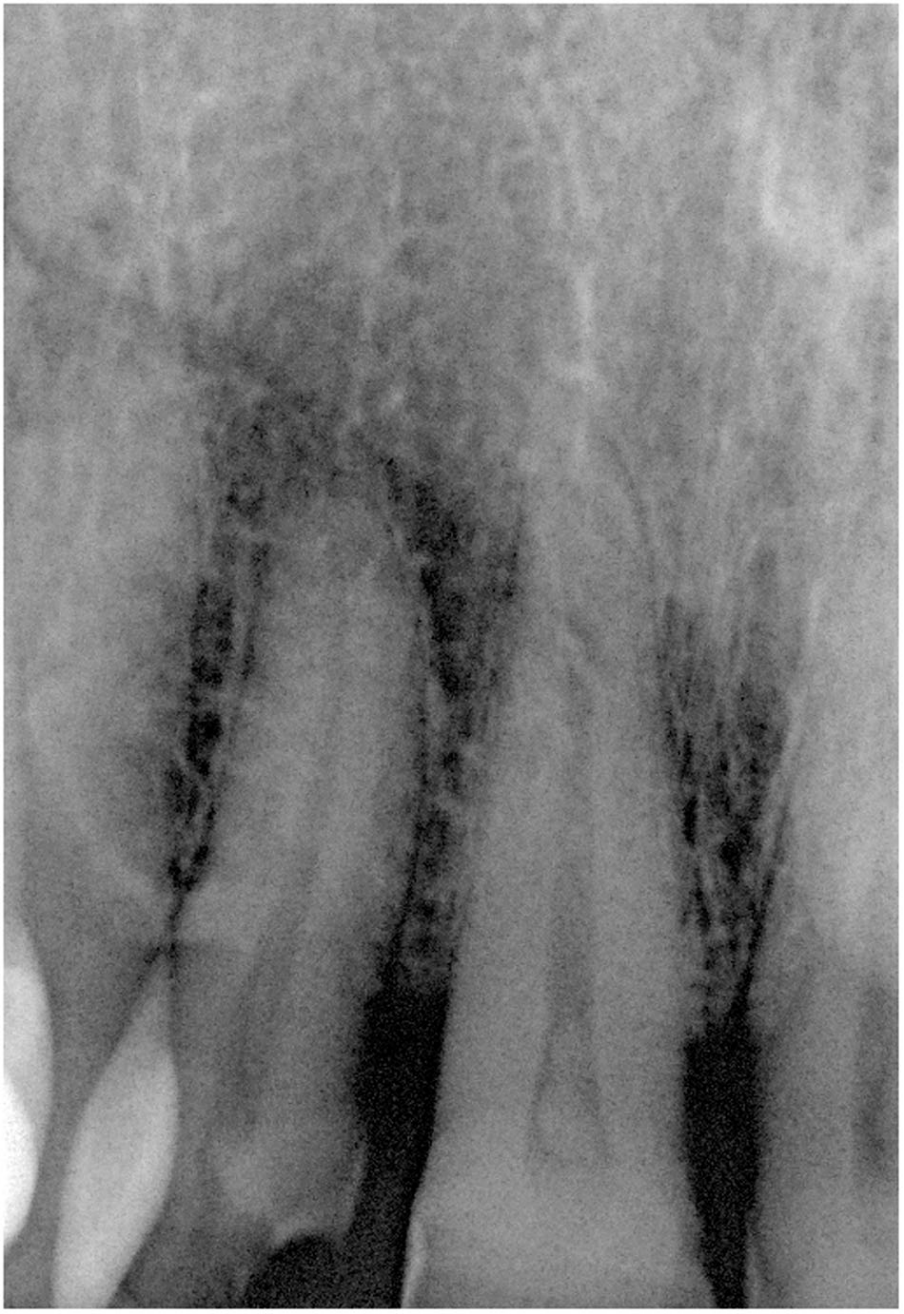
X-ray showing no involvement of the underlying bone
